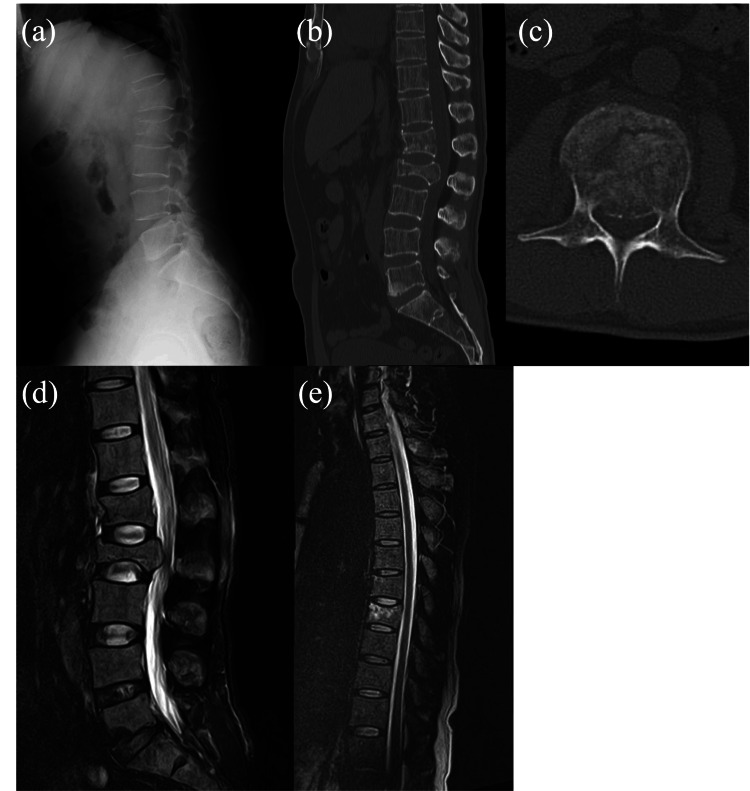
X-ray, CT scan, and MRI on the day of admission. Imaging from the day of admission. (a) X-ray of the thoracolumbar spine. (b, c) CT scan showing axial and sagittal views with severe spinal canal stenosis caused by an L2 burst fracture and an L1 compression fracture. (d, e) MRI of the thoracic spine with a T2-weighted image showing signs of T7 compression fracture.
